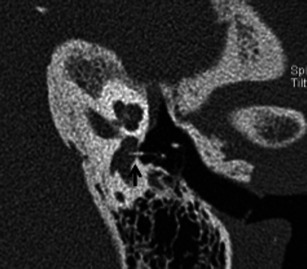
Para-axial HRCT image of the left temporal bone in a patient with severe vertigo, post stapedectomy. The stapes prosthesis is dislocated and lies partly within the vestibule (*arrow*)
